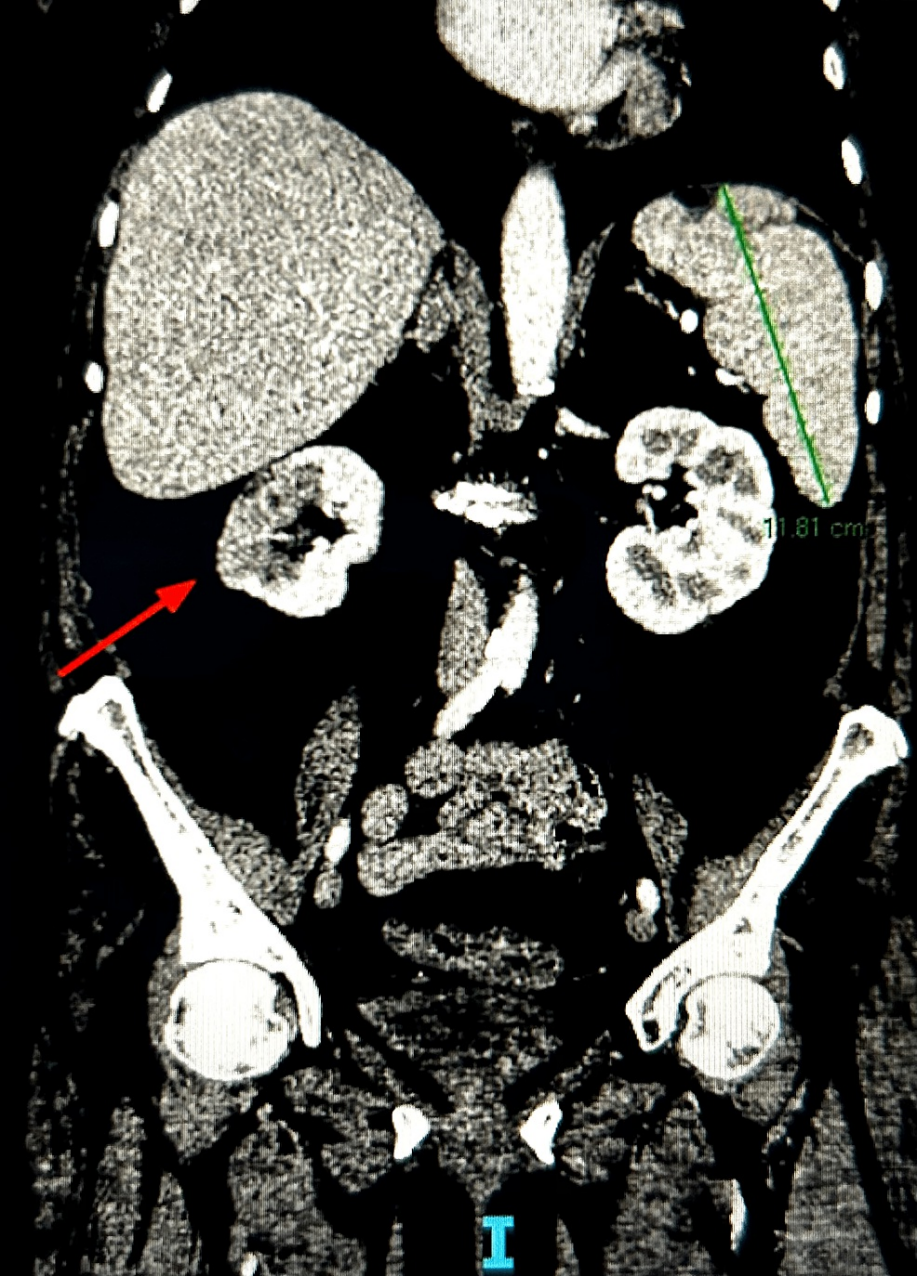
Contrast-enhanced computed tomography of the abdomen and pelvis with the red arrow indicating right wedge-shaped renal infarction.
